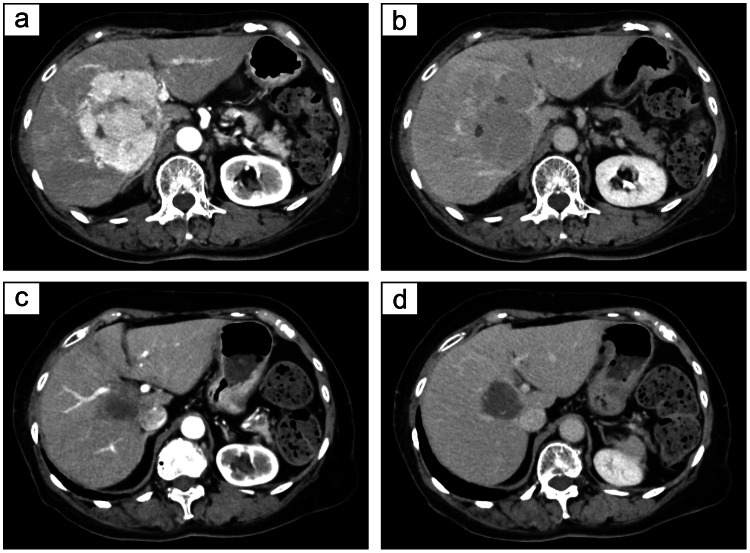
Contrast-enhanced CT scan before and after treatment (a, b) Contrast-enhanced CT scans showing an 8 cm mass in segment 6 of the liver, with a portion of the lesion exhibiting viable tumor tissue. (c, d) Follow-up contrast-enhanced CT showing a reduction in the segment 6 liver mass, with the previously noted viable portion no longer visible.
